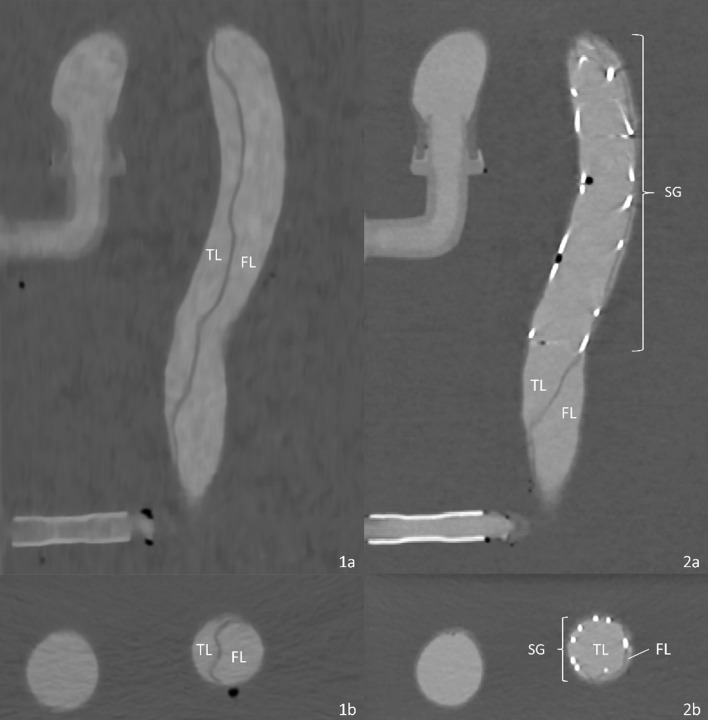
CTA imaging before (1a, b) and after TEVAR (2a, b) TL: True Lumen, FL: False Lumen, SG: Stentgraft
